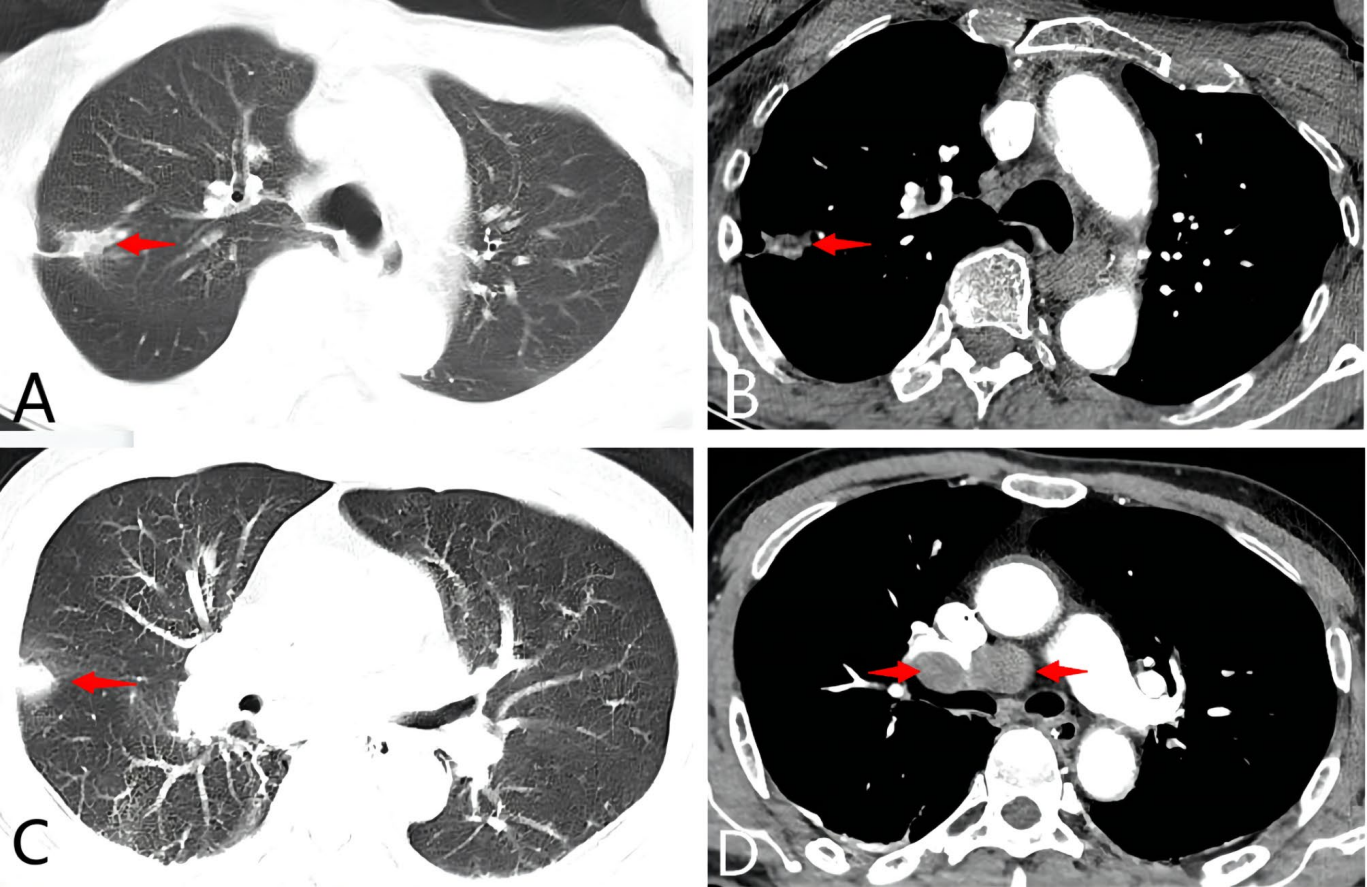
Enhanced chest CT changge of partial patients. Enhanced chest CT showed a nodule in the upper right lung in patient 6 (A, B); enhanced chest CT showed bilateral pulmonary multiple nodules in patient 9 (C), with multiple markedly enlarged lymph nodes in the right hilum (D)
